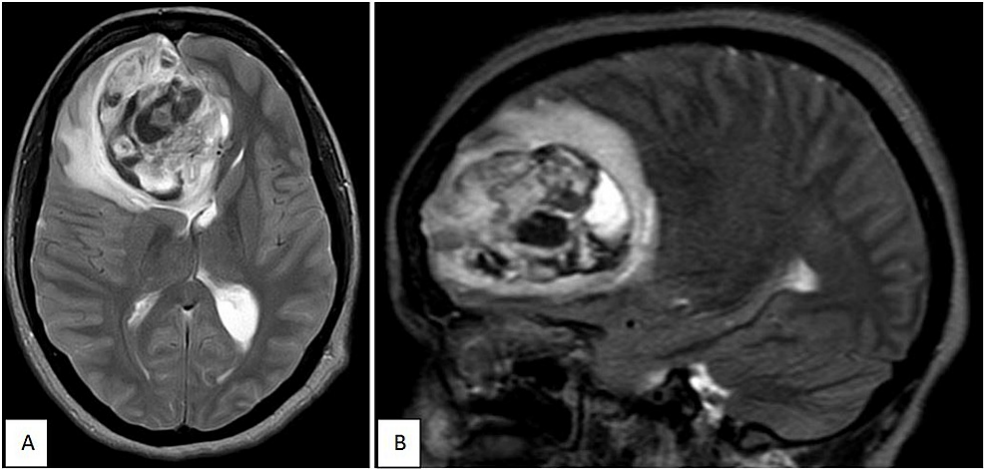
MRI Brain (T2W) - 6.5 x 5.0 cm heterogeneous, T2 hyperintense lesion in the right frontal lobe, involving and effacing surrounding parenchyma, corpus callosum with mild perilesional oedema. There was a midline shift of 10 mm to the left.
